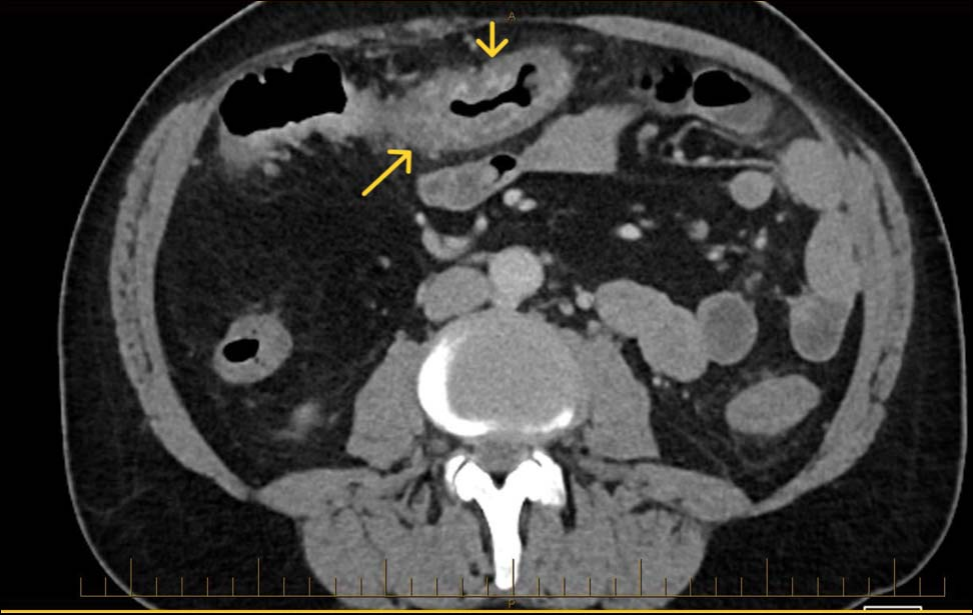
CT axial view showing colitis. Arrows showing colon wall thickening/oedema likely reflecting varices.
